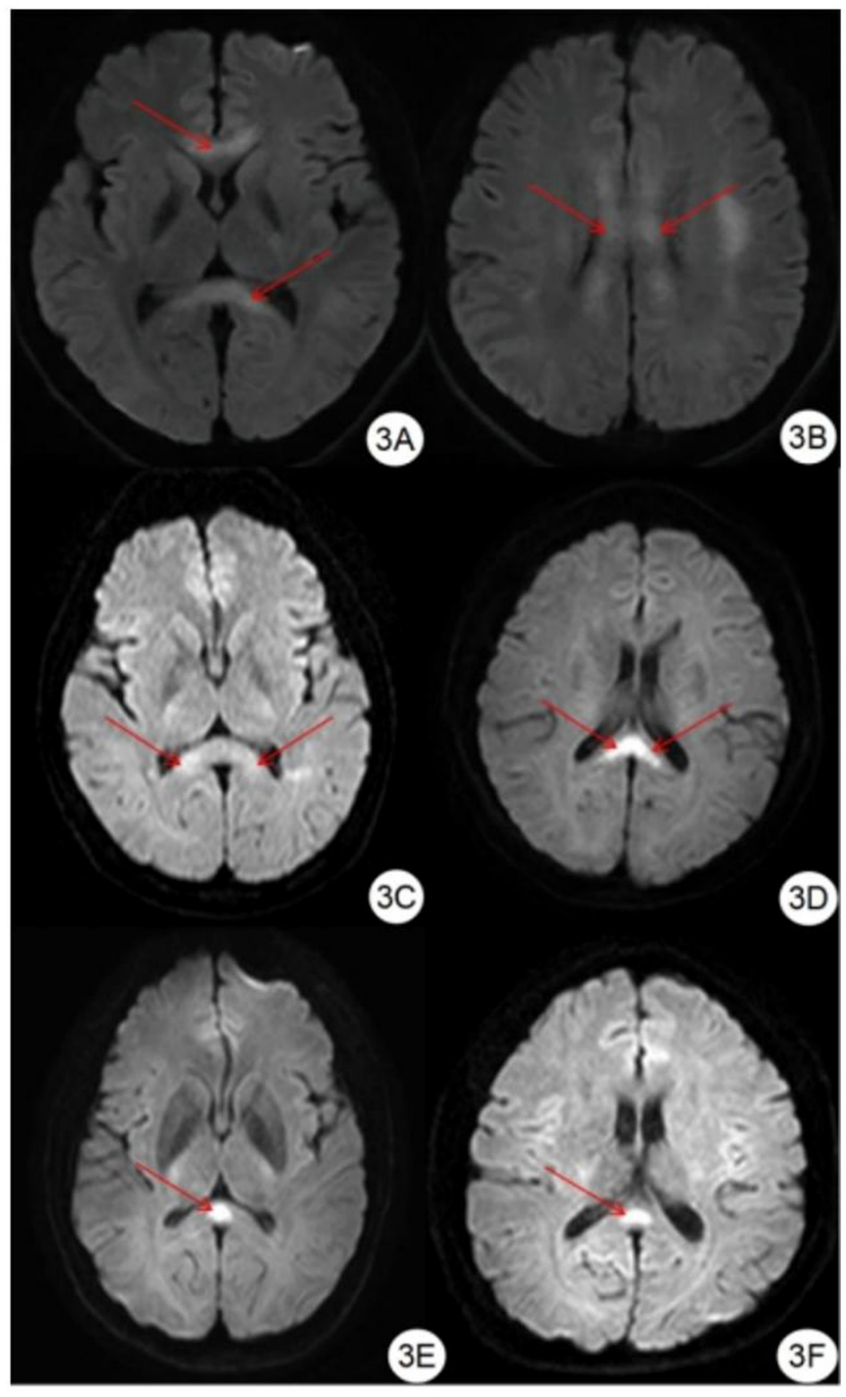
Classification of edema in corpus callosum region. (A, B) DWI showed symmetrical hyperintensity in the splenium, trunk, and genu of the corpus callosum. (C, D) DWI showed symmetrical hyperintensity in the splenium of the corpus callosum. (E, F) DWI showed hyperintensity in the center of the splenium of the corpus callosum.
